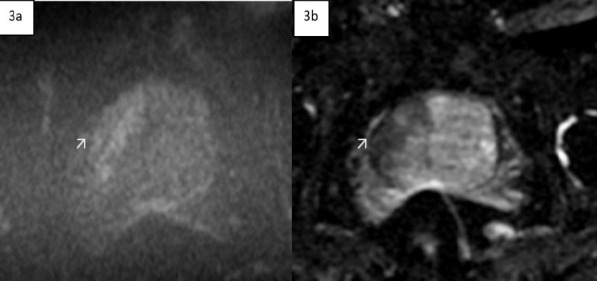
The lesion shows evidence of diffusion restriction on the DWI (3a) and ADC mapping 3b
